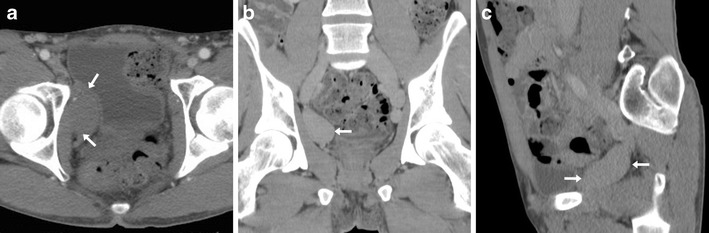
Post-thrombotic aneurysmal dilatation of the right hypogastric vein in a 34-year-old male intravenous drug user with long-standing history of lower limb thrombophlebitis. Requested to investigate sepsis and suspected deep venous thrombosis, CT showed a well-demarcated ovoid mass lesion abutting the right obturator muscle, measuring 30–35 HU precontrast attenuation (A). The lesion compressed the urinary bladder, enhanced homogeneously and synchronously with venous structures (B, C). Surgical repair was unnecessary considering absence of complications [Adapted from Open Access ref. no. [40]]
